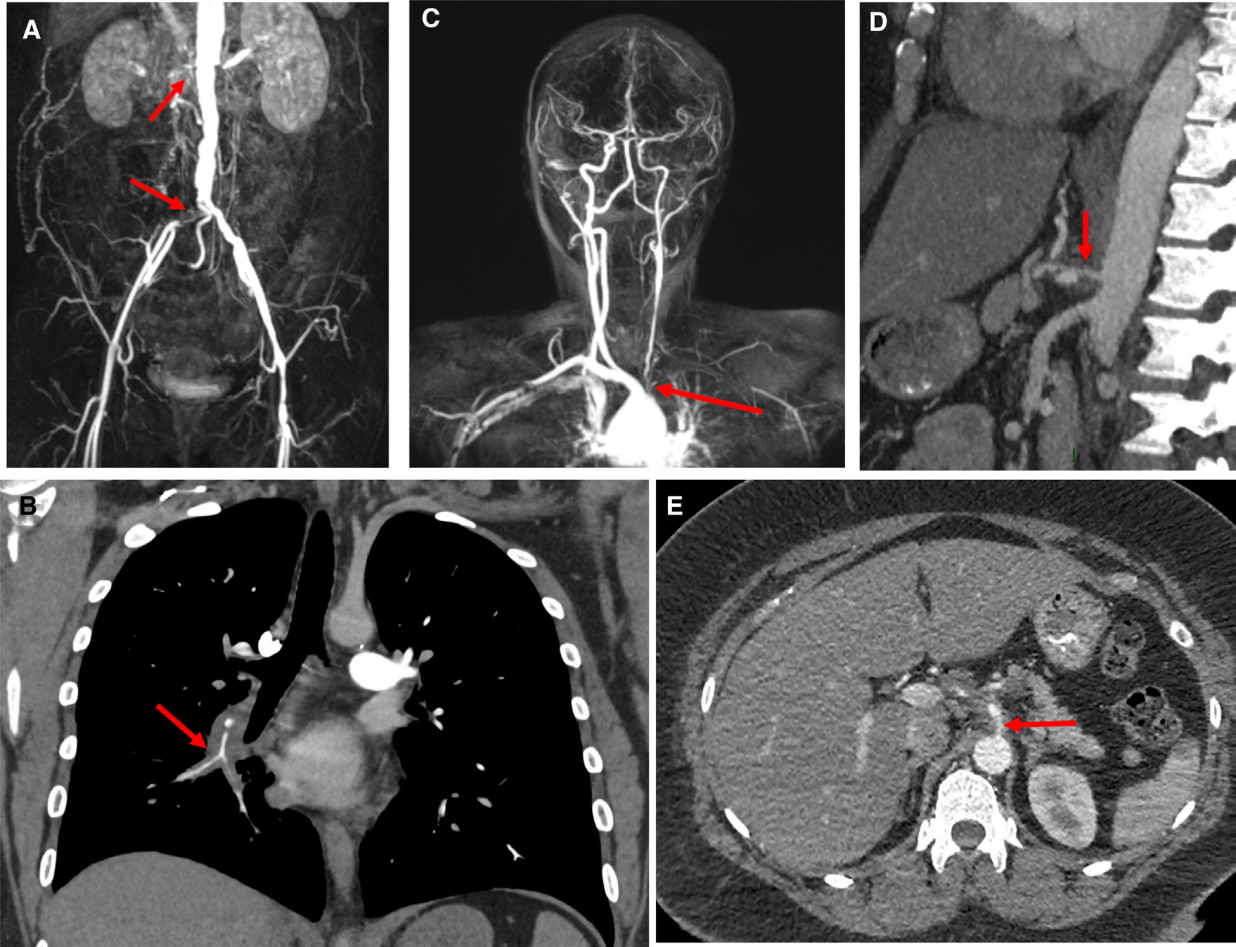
Examples of vascular stenosis and occlusion in Takayasu arteritis. A, Magnetic resonance angiography (MRA) showing occlusion of the right renal artery and right iliac artery. B, A computed tomography (CT) pulmonary angiogram showing thickening around a stenotic portion of the right lower lobe pulmonary artery. C, MRA showing stenosis of the left common carotid artery and proximal occlusion of the left vertebral and subclavian arteries. D and E, Same contrast-enhanced CT showing stenosis and probable dissection of the coeliac axis with surrounding inflammatory change.
